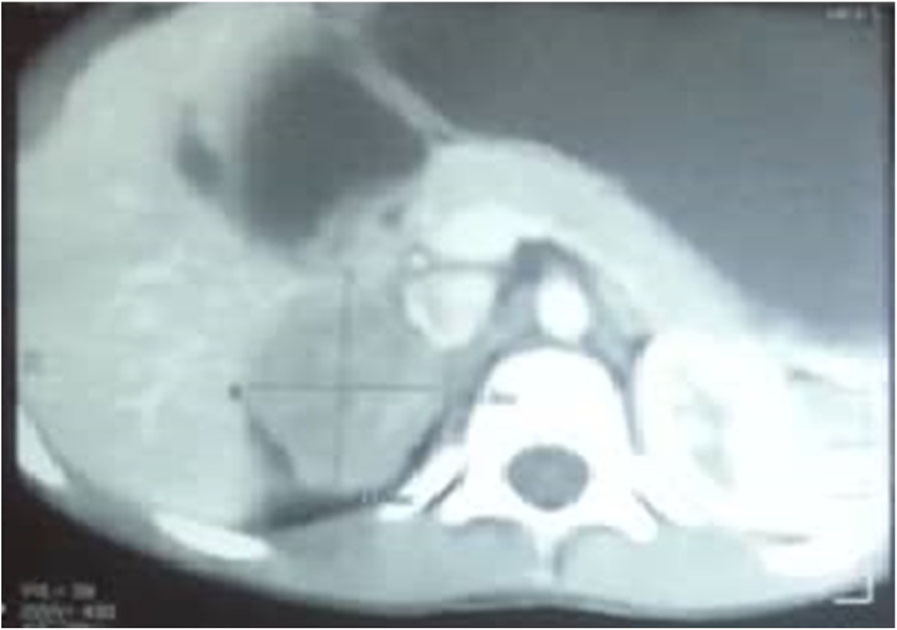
CT scan of the abdomen showing a right adrenal tumor
